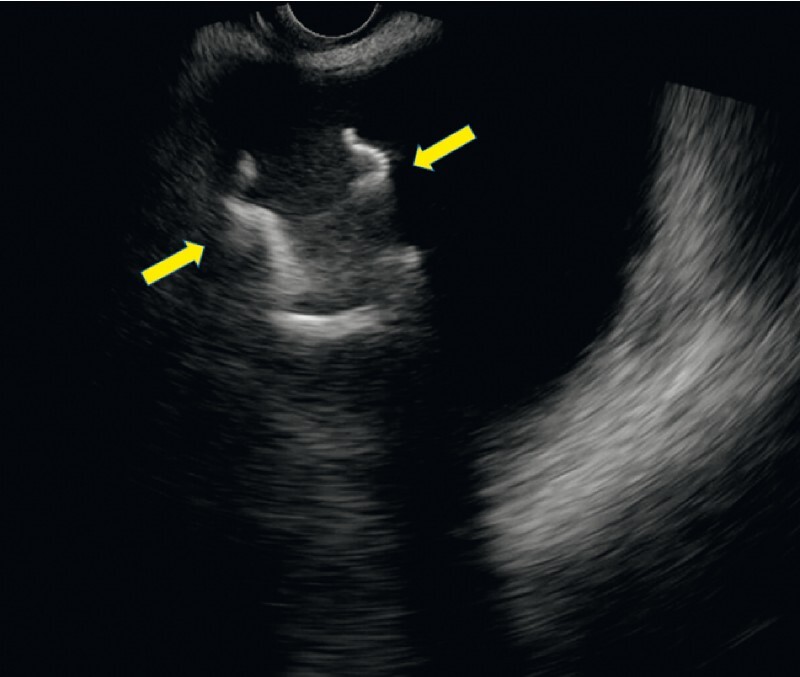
Endoscopic ultrasonography (EUS) shows the migrated lumen-apposing metal stent (LAMS) (yellow arrows) floating in the cavity of the WON.
